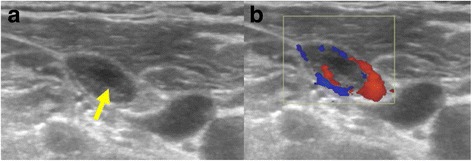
Lymph node metastasis a in a patient with known multifocal papillary carcinoma of the thyroid showing a small area of necrosis (arrow). Color doppler image showing pathological peripheral vascularity b
